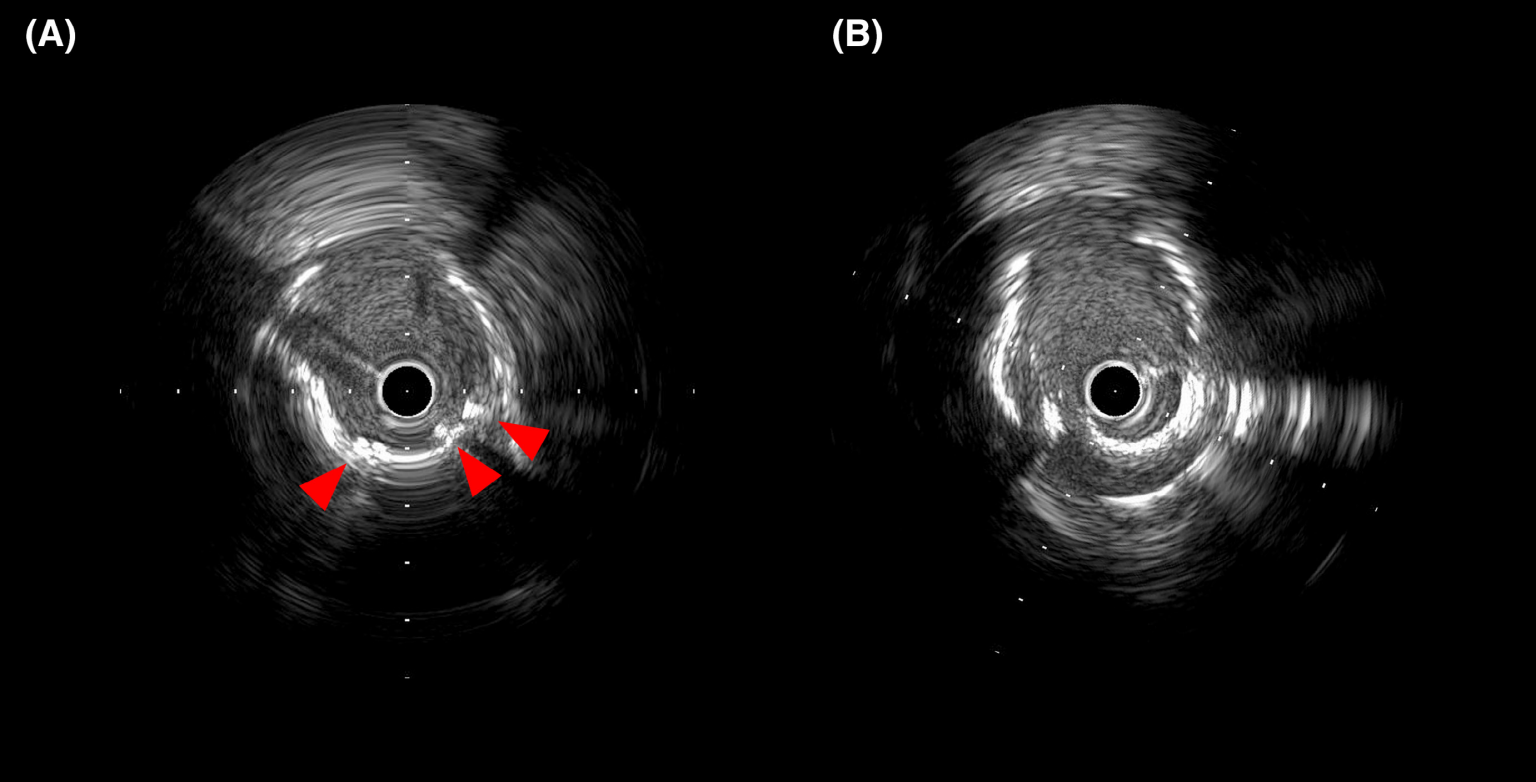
Intravascular ultrasonography at the under‐expanded stent site. (A) Baseline; (B) post‐stentablation with rotational atherectomy (RA). (A)Intravascular ultrasound (IVUS) at the under‐expanded stent site. IVUS findings at baseline suggest inadequate stent dilatation caused by highly calcified lesions (red arrowheads). (B) IVUS after stentablation (SA) with RA. Stent struts have fractured after SA. SA, stentablation; IVUS, intravascular ultrasound.
